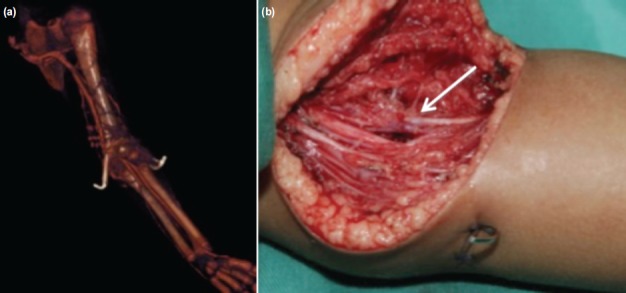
A 2-year old girl was referred with a pink and pulseless hand following a reduction of Gartland III supracondylar fracture; (a) CT angiogram revealed brachial artery block with good distal run-off. (b) Surgical exploration and tissue release were able to restore brachial artery flow and return pulses.
